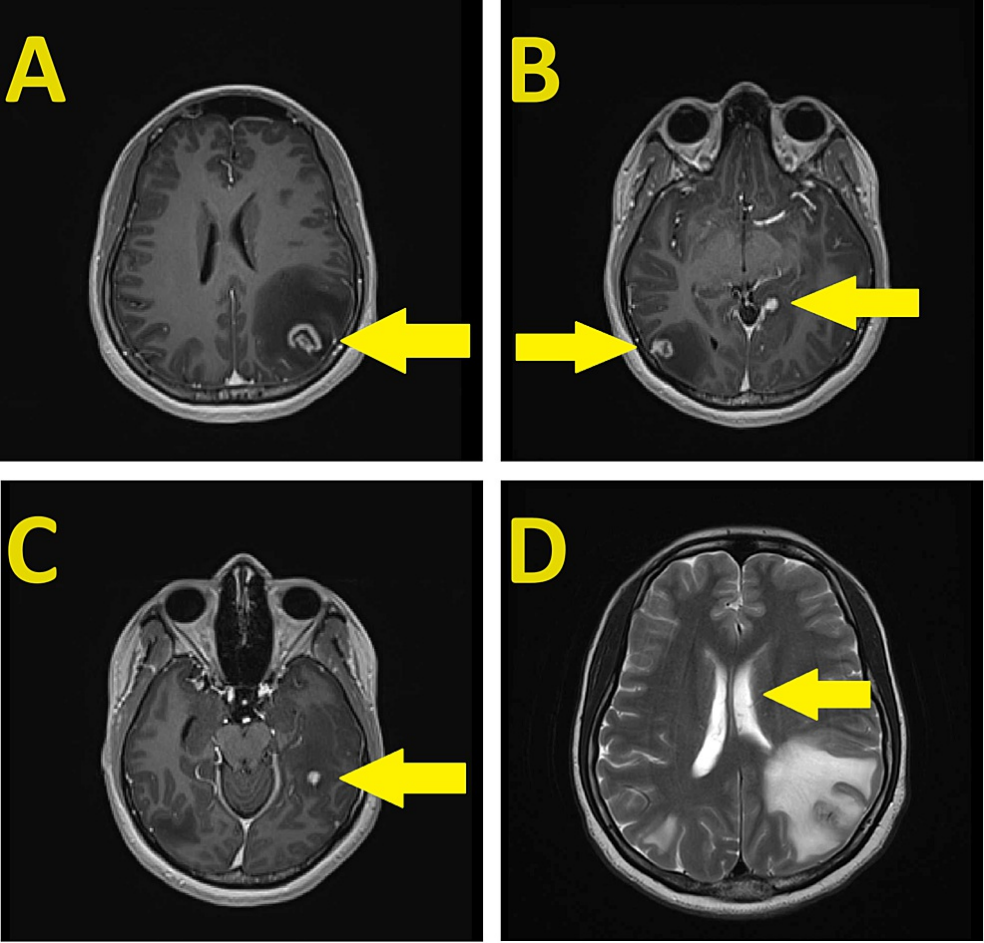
Magnetic resonance imaging (MRI) scans of the brain (A-C) Post-contrast enhanced MRI axial T1-weighted stealth sequences showing four contrast-enhancing lesions surrounded by vasogenic edema. The lesions are located in the left parietal lobe (A), right occipital lobe and left occipital lobe (B), and left temporal lobe (C). The largest lesion measures 1.65 x 1.79 cm (A). (D) An axial T2 MRI image showing a 4-5 mm left-to-right midline shift of the patient.
